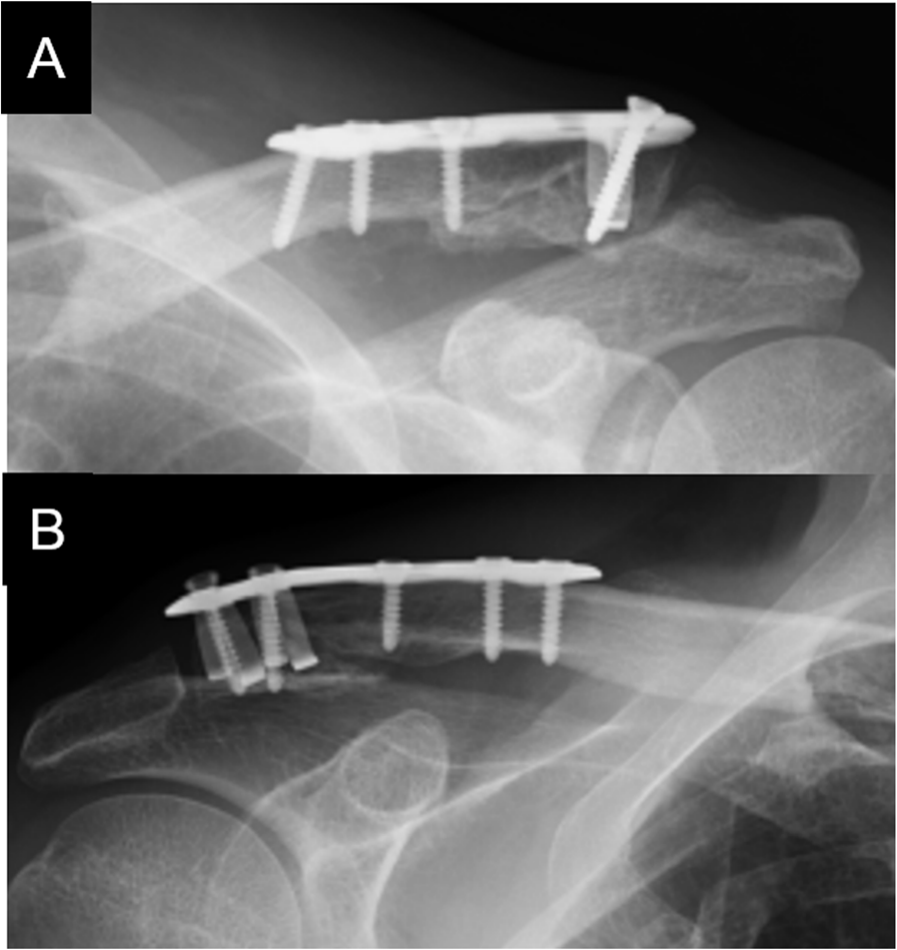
Plain radiographs showing delayed bone union. Post-operative radiographs performed 1 year after surgery revealed that the fracture line is visible and the distal screws were loosening (a and b)
